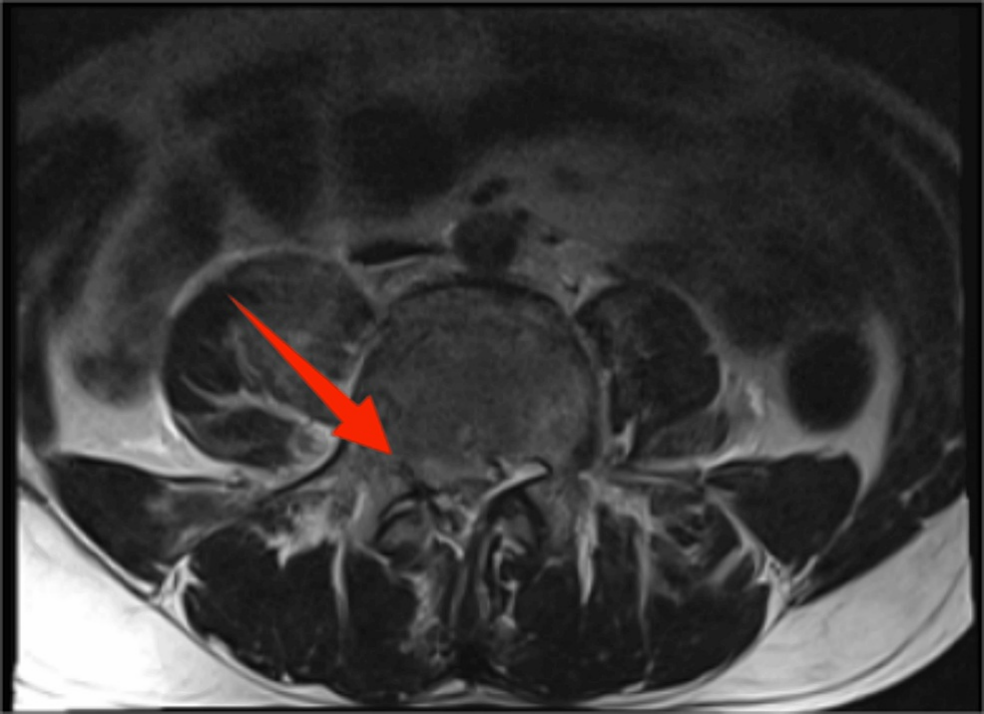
MRI axial image showing L4 vertebral level MRI, magnetic resonance imaging
